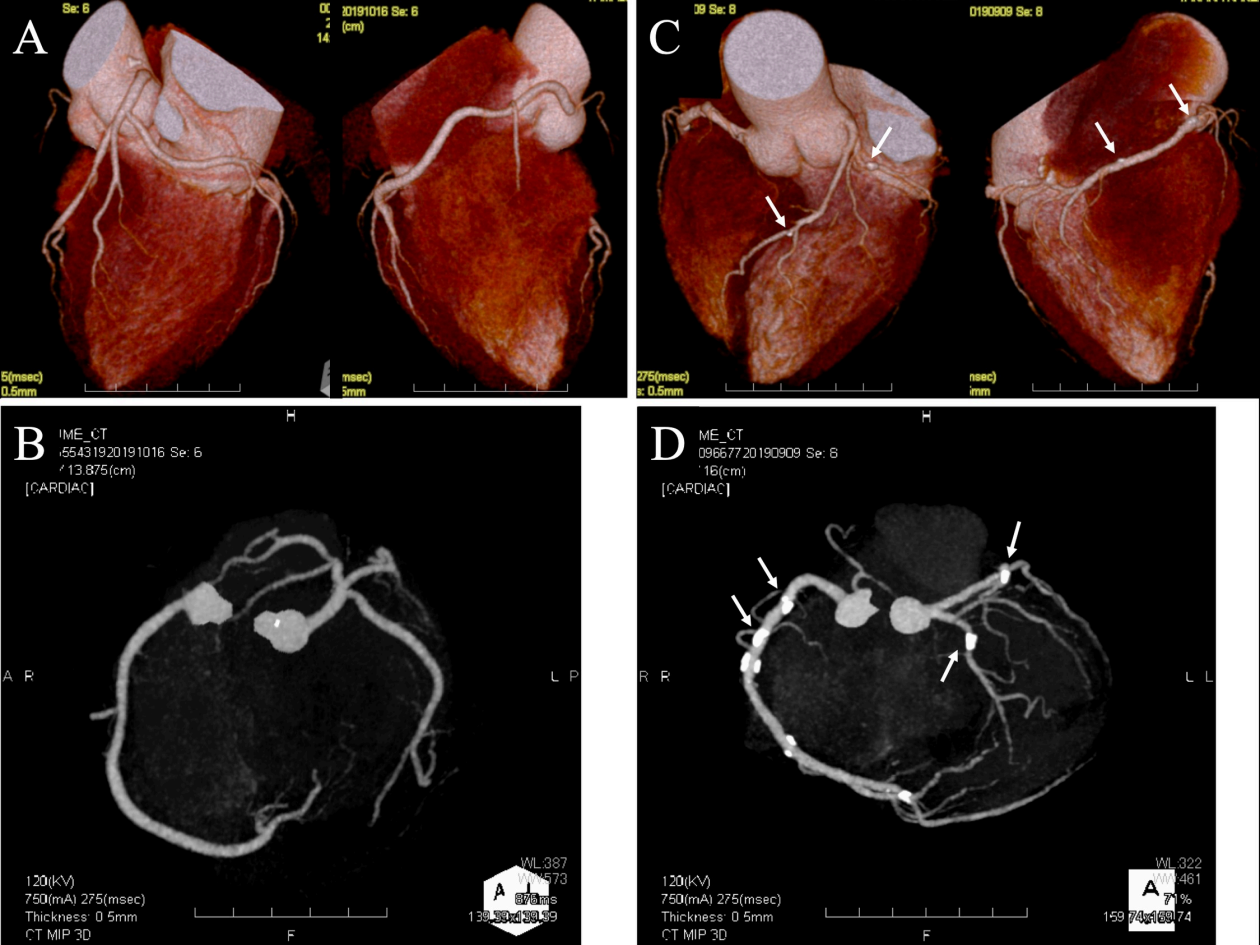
Representative case. (A) Three-dimensional (3D) reconstruction and (B) axial computed tomography image showing no coronary artery calcification (CAC). (C) 3D reconstruction and (D) axial image showing CAC; #1, #2, #6, and #11 are highly calcified. The number of CAC sites was 4 (arrow).
